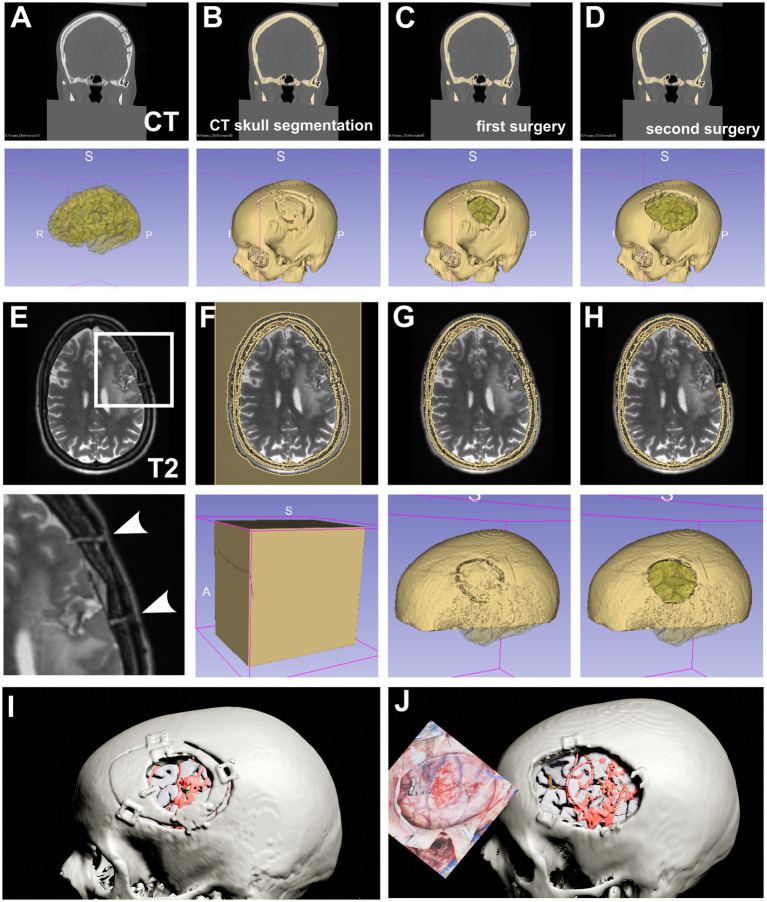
Craniotomy reconstruction from postoperative CT for IP35. (A–C) Initial skull segmentation using the threshold function to isolate hyperdense bone with manual threshold adjustment (top), and resulting 3D skull reconstruction (bottom). Reconstruction of the first craniotomy. Craniotomy boundaries were identified as subtle hypodense lines on CT, and the skull flap was manually removed using slice-by-slice editing to generate the craniotomy model. (D) Reconstruction of the second craniotomy using the thresholding and manual editing approach described in (A–C). (E) Axial postoperative T2 MRI slice (top) with zoomed-in view and arrowheads pointing to postoperative evidence of the craniotomy. (F–H) Initial skull segmentation using inverse thresholding to isolate hypointense skull regions with manual threshold adjustment, and resulting 3D reconstruction. The skull segmentation was manually refined slice-by-slice in 3D Slicer (Eraser and Scissors tools) to remove artifacts and improve skull boundaries, producing a cleaned 3D skull surface, with artifact removed using slice-by-slice editing to generate the final craniotomy model. (I,J) Blender files of the resulting craniotomy with vasculature and brain reconstruction for the first and second surgery.
